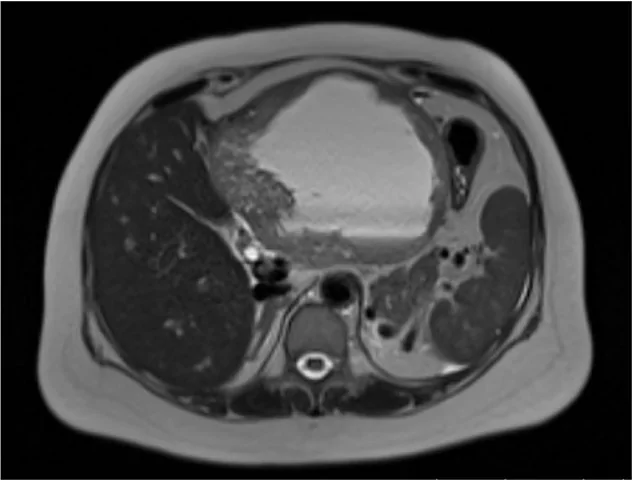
MRI scan showing a thick-walled cystic lesion [14.0 × 12.7 × 9.8 cm] inseparable from the left lobe of the liver.
